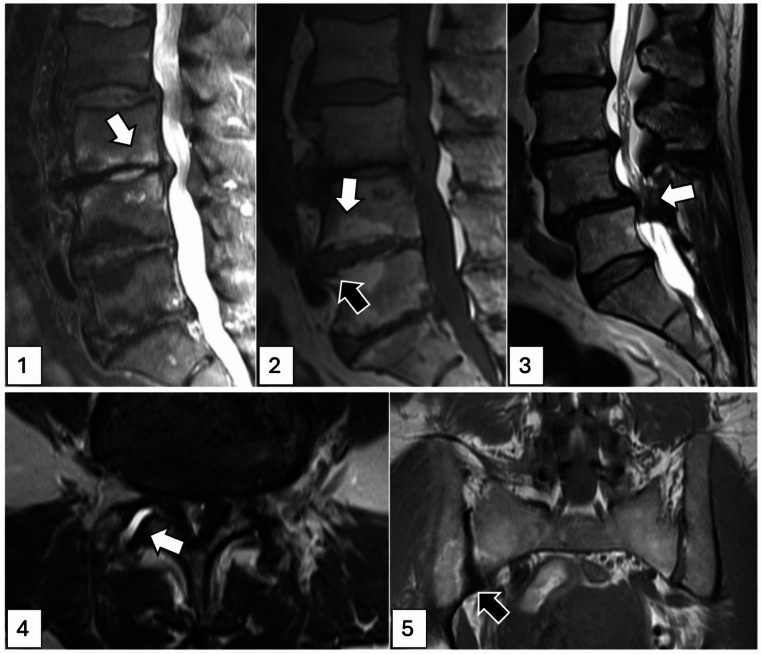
Imaging lesions. 1 = Mid-spinal section of sagittal T2 weighted MR image with fat saturation, showing Modic type 1 lesions (white arrow) in the L3/4 disc segment. 2 = Mid-spinal section of sagittal T1-weighted MR image, showing Modic type 2 lesions (white arrow) and endplate erosion (black arrow) in the L4/5 disc segment. 3 = Mid-spinal section of sagittal T2-weighted image without fat-saturation, showing severe central canal stenosis (white arrow) in the L4/5 disc segment. 4 = Axial T2-weighted image without fat-saturation, showing facet arthropathy with fluid in the right facet joint (white arrow). 5 = Coronal T1-weighted image showing degenerative SI joint changes on the right side with sclerosis (black arrow)
